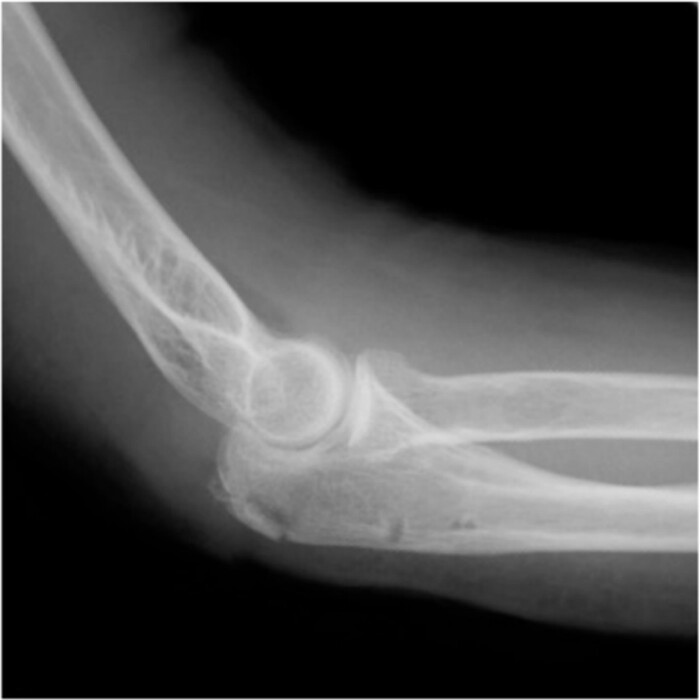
Postoperative lateral elbow radiographs. The bone fragment is reduced and fixed.
